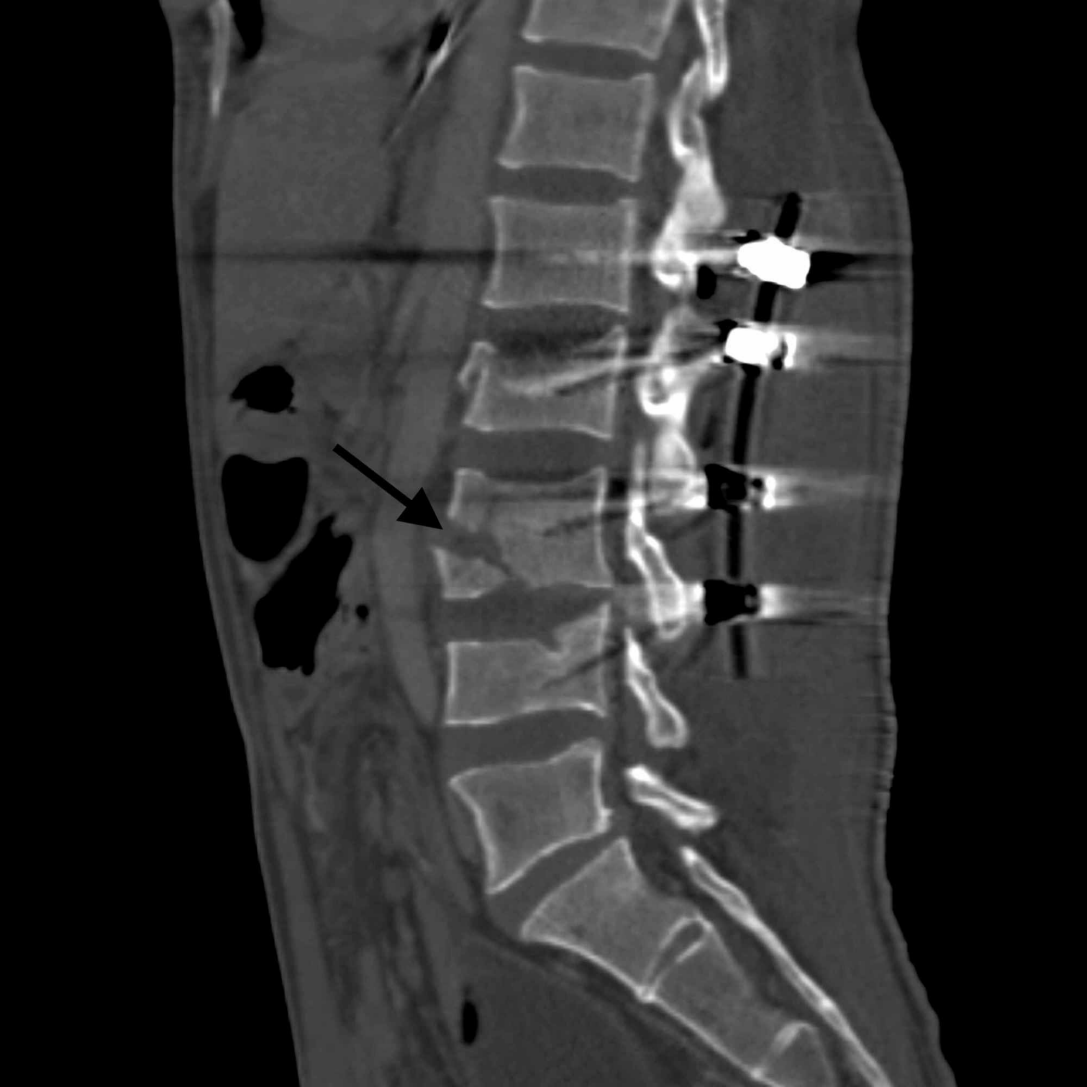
Postoperative sagittal CT demonstrating a slight widening of the ventral defect at L3.
